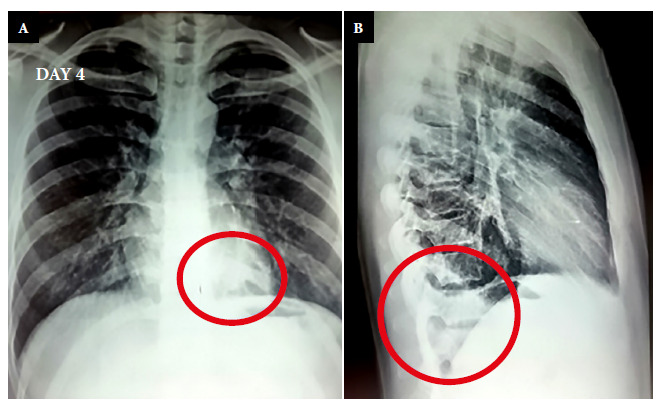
Posteroanterior and lateral chest radiographs on day 4 of the disease. (A) An opacity can be found in the anteroposterior face at the left paravertebral level (circle). (B) An opacity can be found in the lateral face at the level of the last dorsal vertebrae that blocks the costodiaphragmatic angle on that side (circle).
